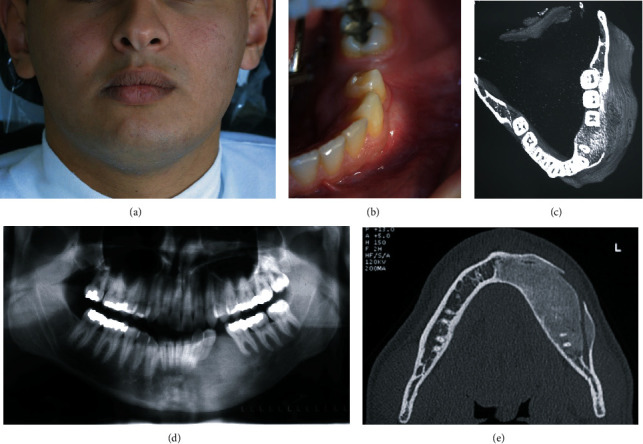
(a) Facial asymmetry at 10 years. (b) Clinical view of the intraoral swelling. (c) Panoramic X-ray at 10 years. (d) Axial image of a CBCT showing the expansion of the lesion to the midline and discontinuity of the buccal cortex. (e) 3D reconstruction showed an increase in the volume of the left hemimandible.
